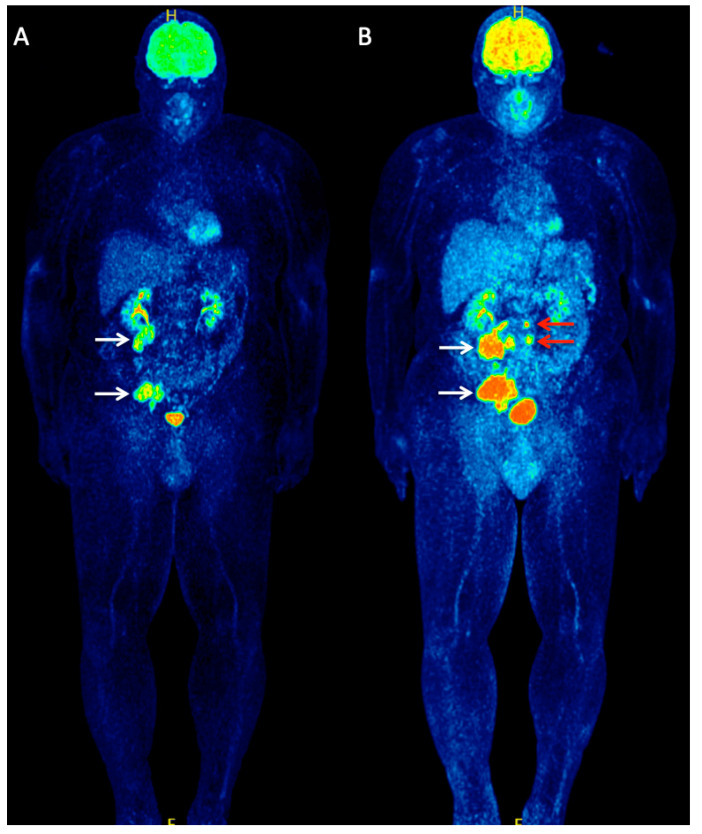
A 56-year-old patient with metastatic MCC of unknown primary referred to our department for staging purposes before initiation of immunotherapy. Whole-body ^18^F-FDG PET (MIP) (A) demonstrated two large hypermetabolic lesions in the abdomen and pelvis, corresponding to lymph node metastases (white arrows). The patient received treatment with the cytotoxic T-lymphocyte-associated protein 4 (CTLA-4) inhibitor, ipilimumab, considered at the time of scanning as a potentially beneficial immunotherapeutic agent for MCC. After receiving two cycles of ipilimumab, the patient underwent an interim follow-up ^18^F-FDG PET/CT for early treatment response evaluation. Whole-body ^18^F-FDG PET (MIP) (B) revealed a clear disease progression with an increase in size and metabolism of the previously observed metastases (white arrows) but also detected newly appeared metastatic retroperitoneal lymph nodes (red arrows).
